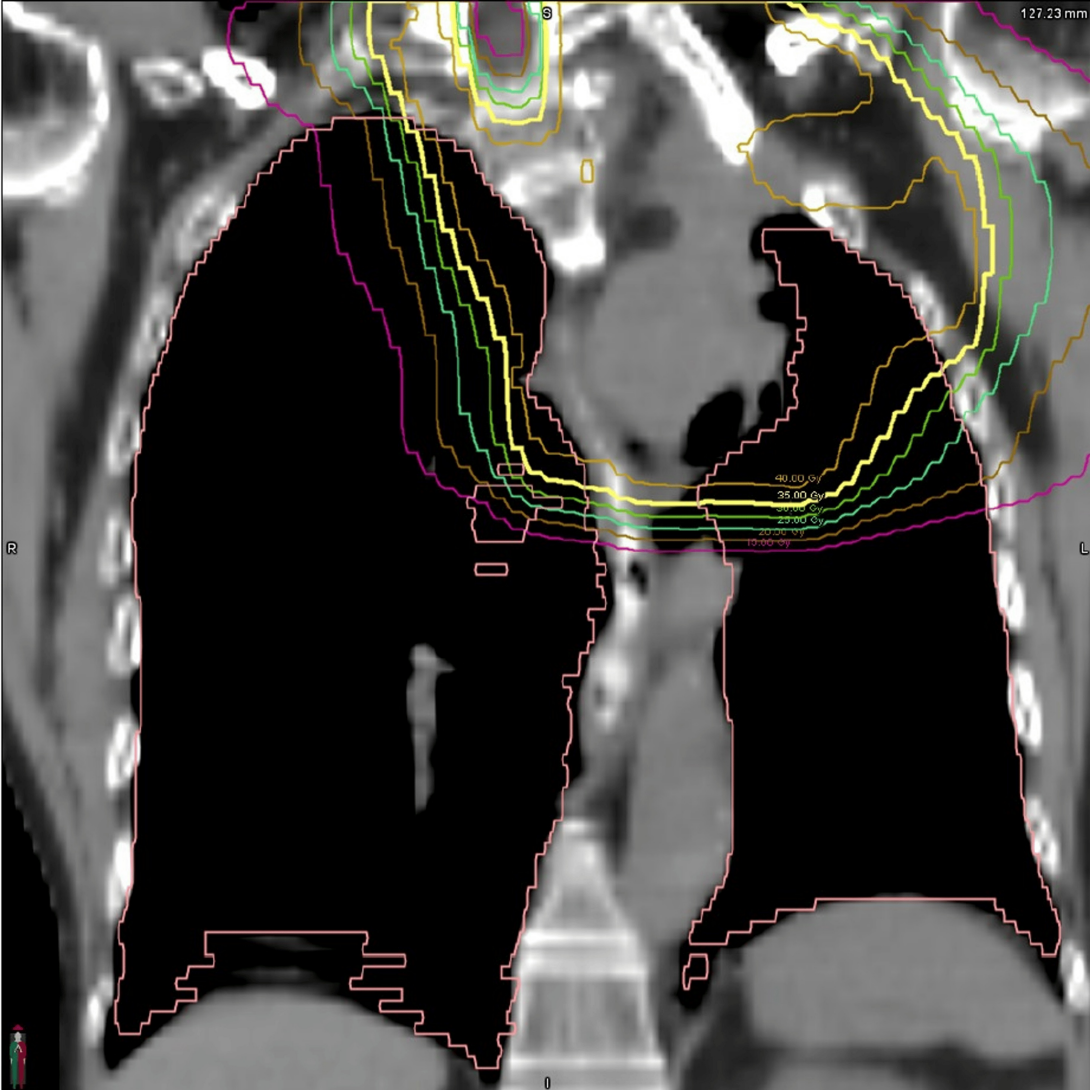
Palliative reirradiation plan targeting the recurrent Pancoast tumor of the left lung Coronal CT view. No GTV is shown. GTV: gross tumor volume
